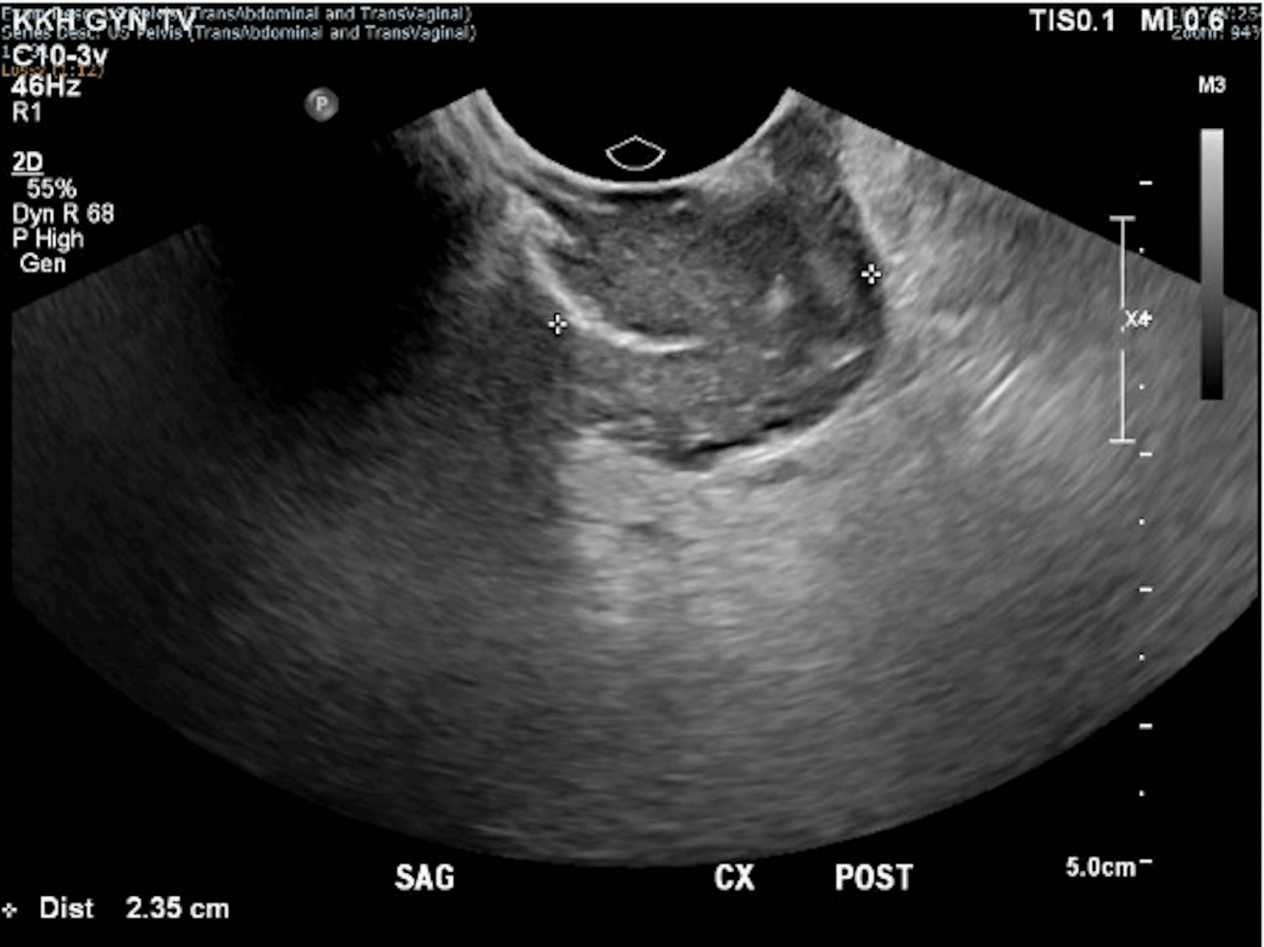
Sagittal view of the posterior cervix on transvaginal ultrasonography showing a heterogeneous structure with increased vascularity measuring 3.5 x 2.4 x 1.9 cm posterior to the cervix.
